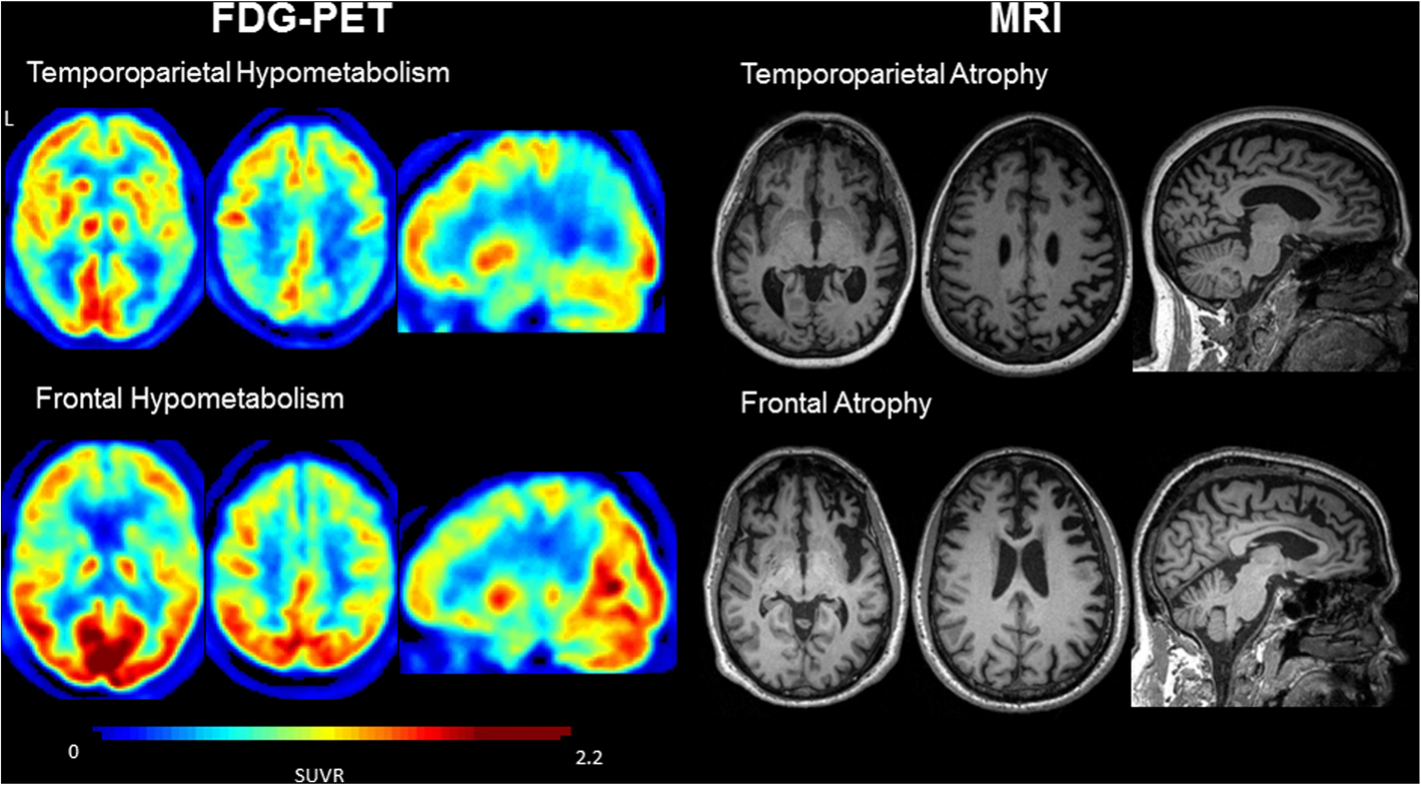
Typical FDG PET metabolic patterns and MRI atrophy patterns. FDG, fluorodeoxyglucose; MRI, magnetic resonance imaging; PET, positron emission tomography.
